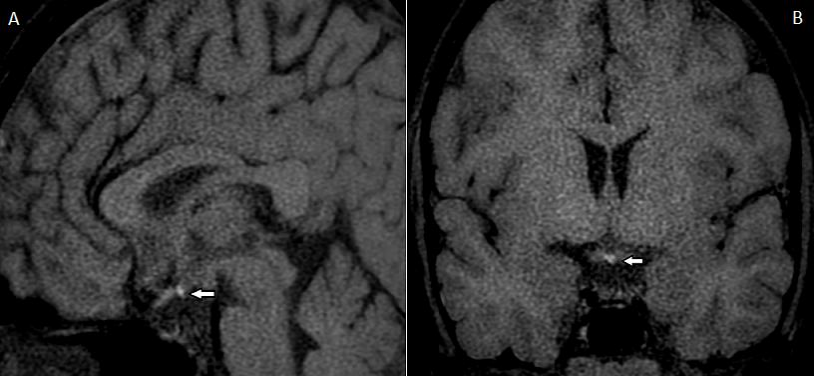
MRI pituitary gland, T1-weight non-contrast sagittal: A) and coronal view; B) showing a shallow sella turcica with the hypoplastic pituitary gland, interrupted pituitary stalk, and an ectopic bright spot site at the infundibular recess (arrow)
